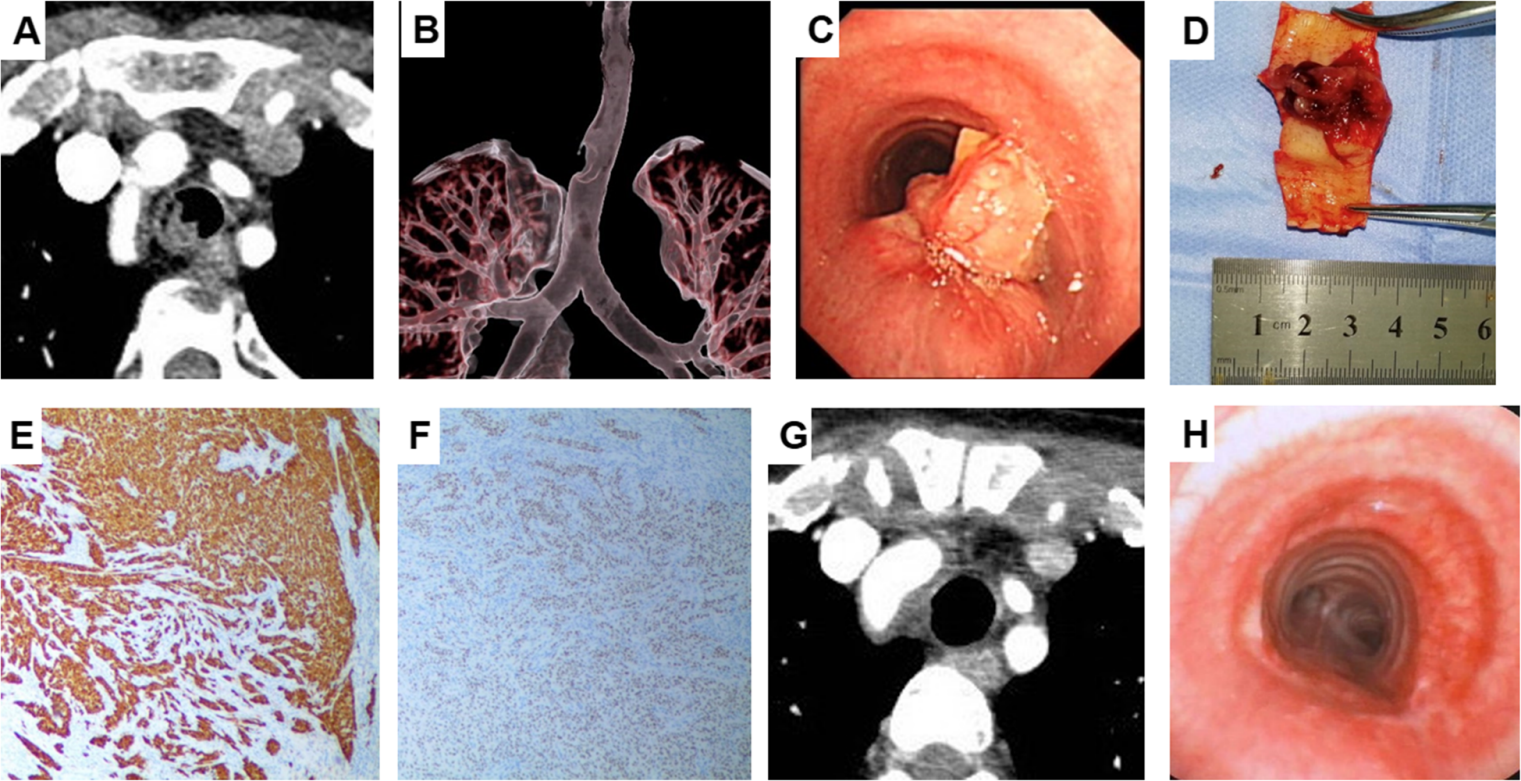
A case of a tracheal malignant peripheral nerve sheath tumour. a Preoperative thoracic CT scans reveal a tumour arising from the right posterior lateral wall of the trachea; b Reconstruction of the airway demonstrated a tracheal defect approximately 4.7 cm above the carina; c Bronchoscopy showed a cauliflower-like mass obstructing 60 % of the tracheal lumen; d View of the resected tumour; e The tumour cells are positive for S100; f The tumour cells are positive for SOX-10; g, h Postoperative thoracic CT scans and bronchoscopy at the six-month follow-up showed no local recurrence
